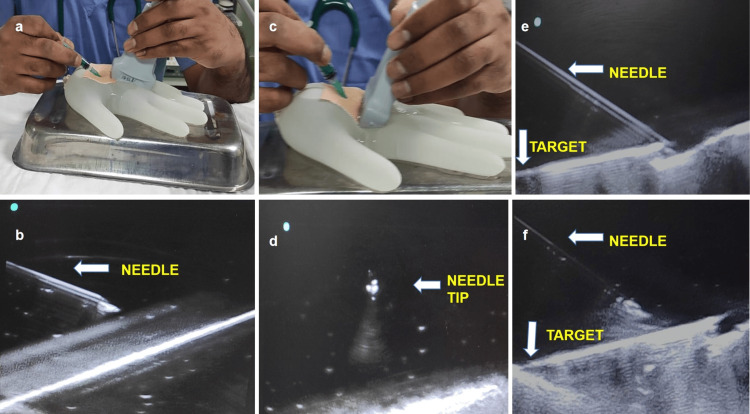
Performance of manoeuvres under ultrasound vision The manoeuvres to be practised are as follows: (a) and (b) In-plane visualisation and advancement of the needle up to the target under ultrasound vision; (c) and (d) Out-of-plane visualisation of the needle tip hitting a target object under ultrasound; (e) and (f) Moving the needle from one end to the other end of a target object under ultrasound vision.
